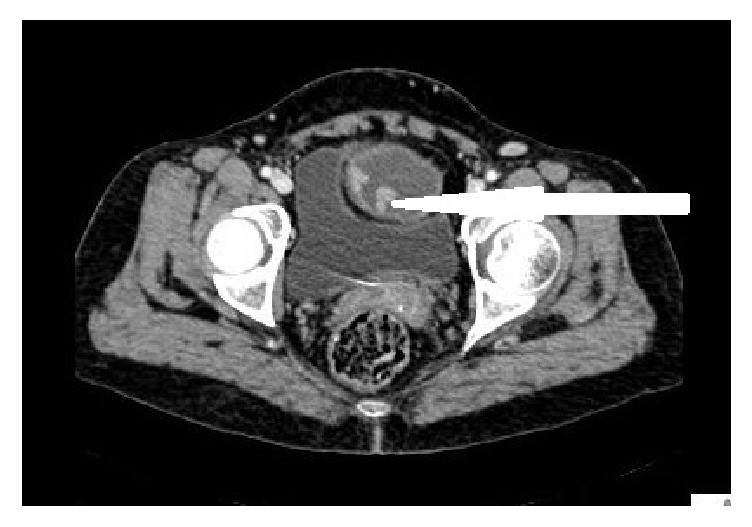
Contrast enhanced computed tomography (CE-CT) image showing cystic right ovary with arrow pointing to enhanced solid densities within the cyst.
